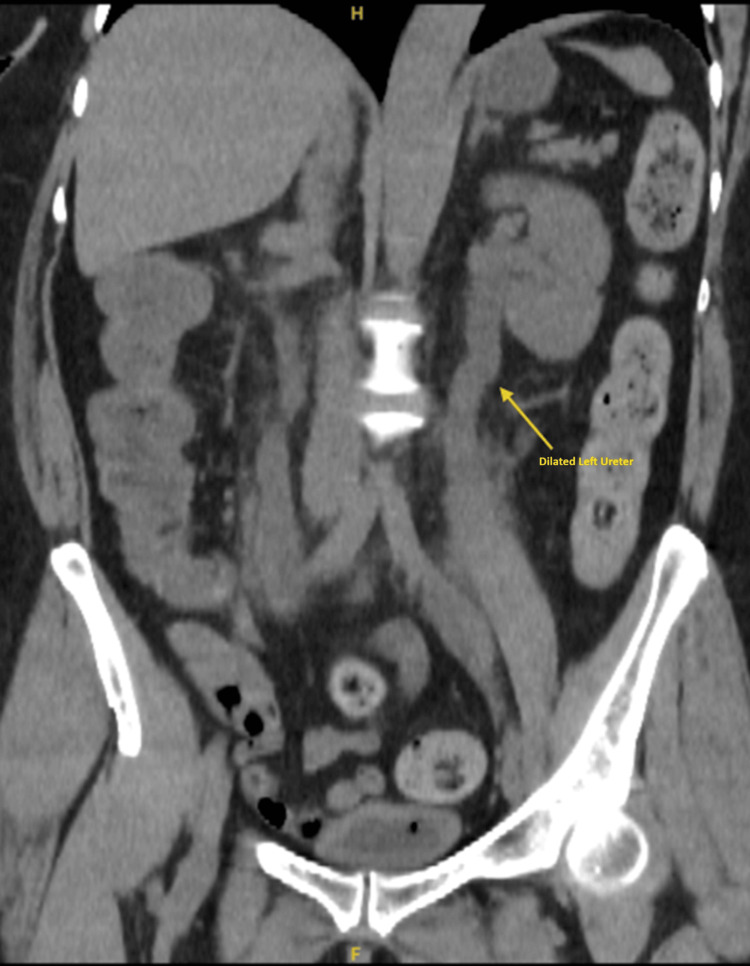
Coronal CT renal tract image demonstrating dilatation of the left ureter (yellow arrow)
